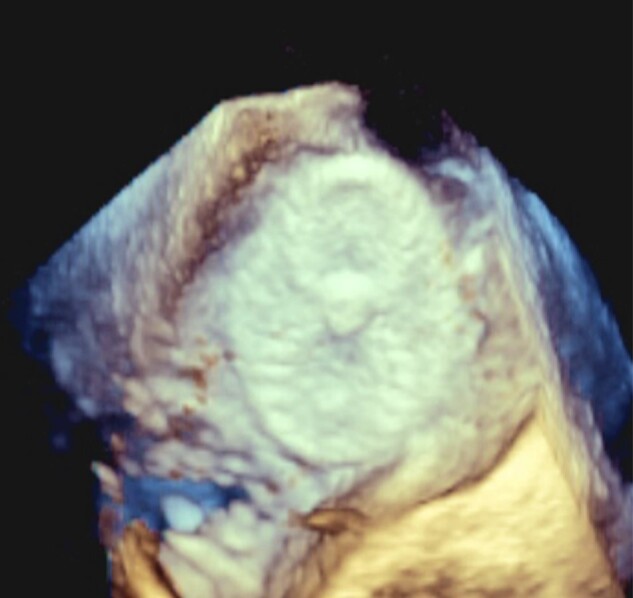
3D echocardiogram, demonstrating endothelium growing over the device which was implanted 7 weeks previously.
